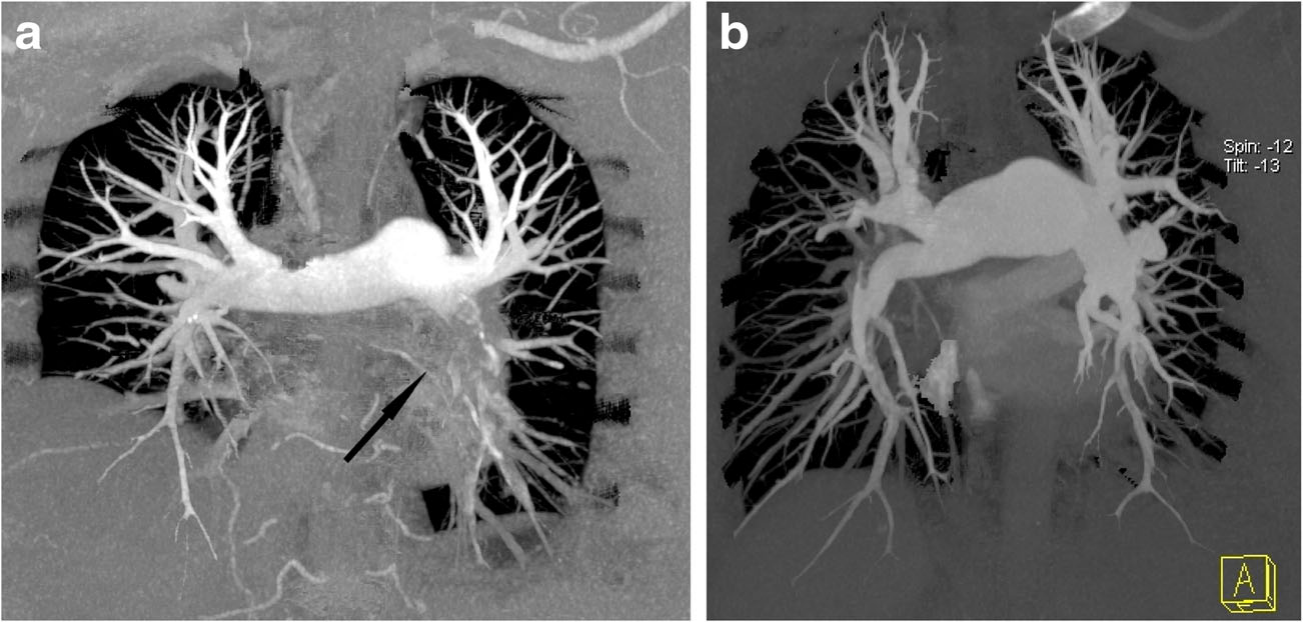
CT pulmonary angiography (coronal plane, MIP reconstructions). a CTEPD: proximal occlusion in the left interlobar pulmonary artery (black arrow) and bilateral segmental and subsegmental vascular obstructions. b CTEPH: bilateral segmental and subsegmental vascular obstructions
